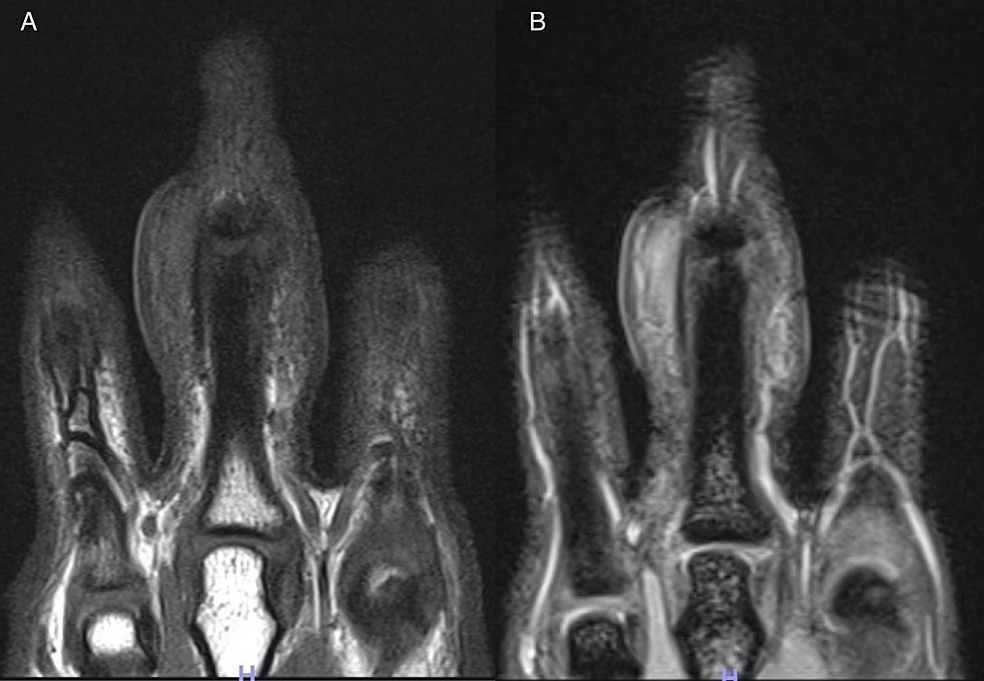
Magnetic resonance imaging Magnetic resonance imaging revealed soft tissue thickening outside of the radial and ulnar collateral ligament of the bilateral third proximal interphalangeal (PIP) joint. The bone cortex, ligaments, and joint space are intact. A) On a T1-weighted image, the lesions were hypointense. B) On a STIR T2-weighted image, the lesions were not as hyperintense.
